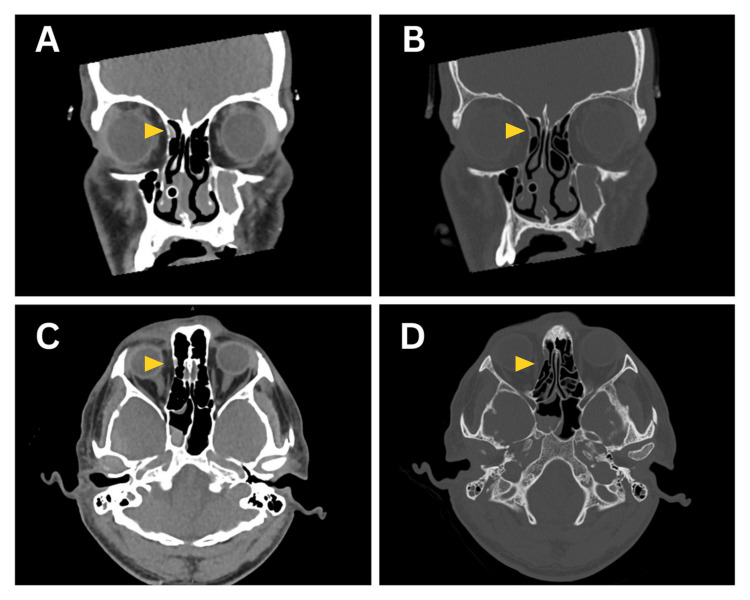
Initial CT imaging of the brain and orbit at presentation. (A-B) Coronal CT sections demonstrate a subtle fracture of the right medial orbital wall (yellow arrowheads) without evidence of medial rectus muscle entrapment. (C-D) Axial CT sections confirm the small medial orbital wall fracture (yellow arrowheads) adjacent to the medial rectus muscle, with preserved extraocular muscle alignment and no radiologic signs of entrapment.
